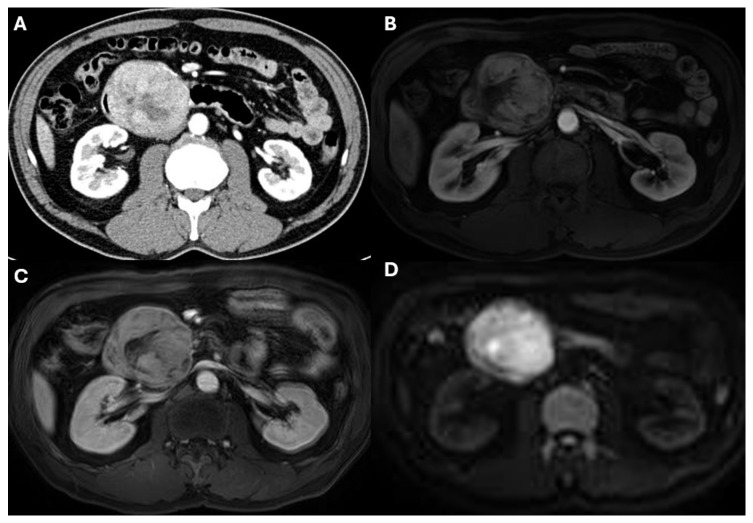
Radiologic assessment of a large pancreatic acinar cell carcinoma. (A) Axial arterial phase CT demonstrates a large, exophytic mass in the pancreatic head with prominent internal low-density areas, suggesting central necrosis. (B,C) Axial T1-weighted MR images obtained before and after Gadovist enhancement. The tumor shows a characteristic hypo-enhancing and heterogenous pattern. (D) Diffusion-weighted imaging reveals marked high signal intensity within the tumor.
